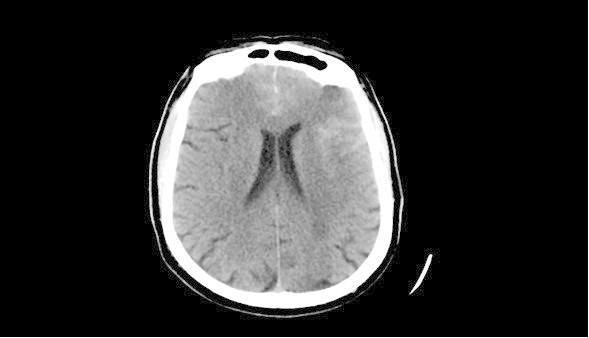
The CT shows widespread hyperdense subarachnoid hemorrhage, within the left frontal sulci and left Sylvian fissure.
